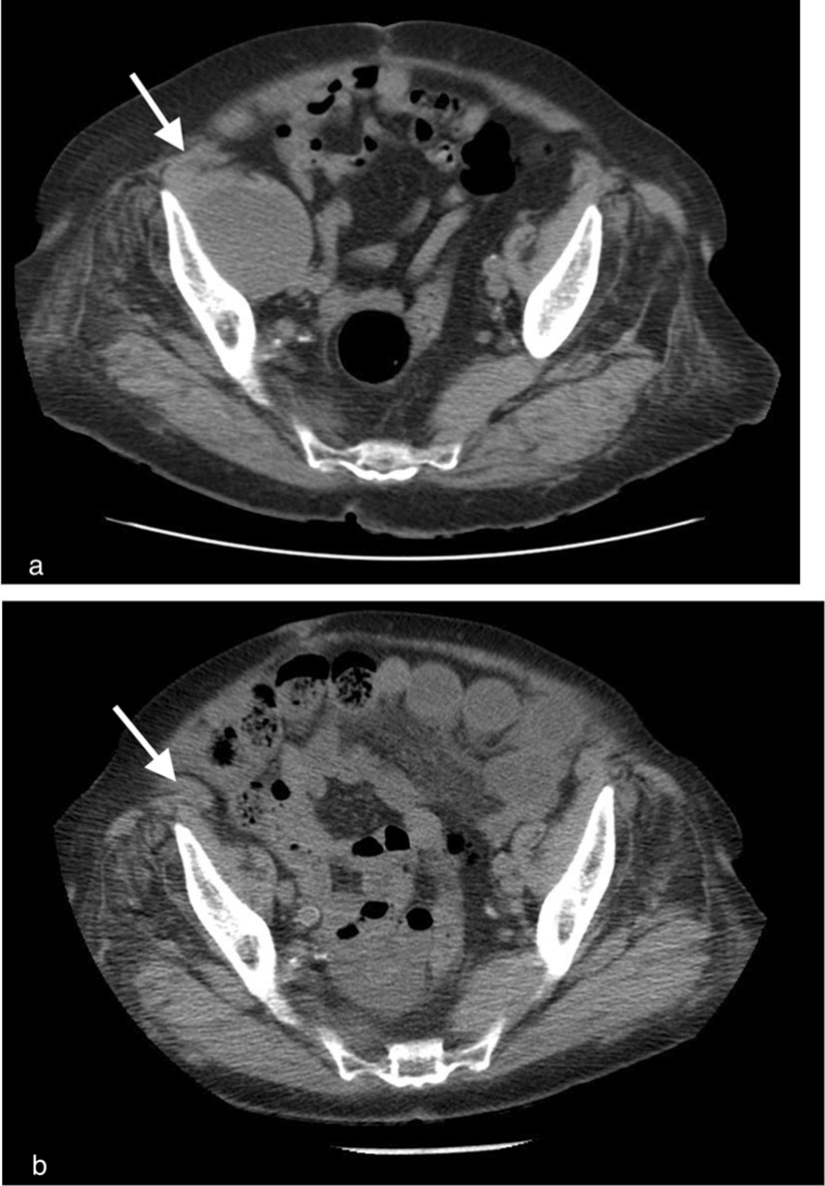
Spontaneous right iliacus retroperitoneal haematoma in a patient on warfarin (a; white arrow) managed conservatively with CT one year later showing complete resolution (b; white arrow)
